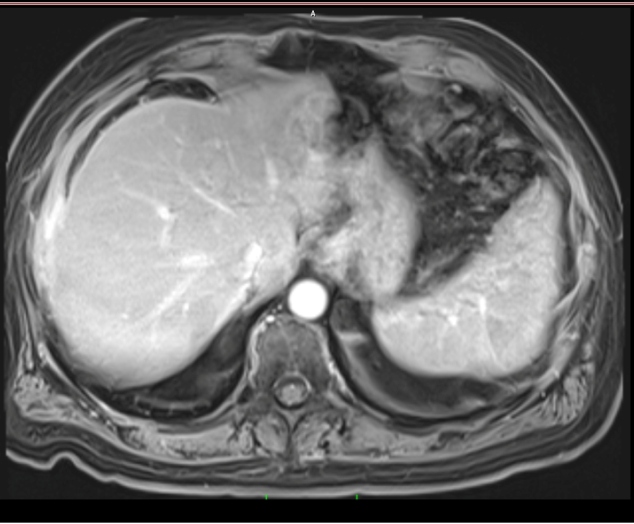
Abdominal magnetic resonance imaging revealed no abnormal findings in the liver.
